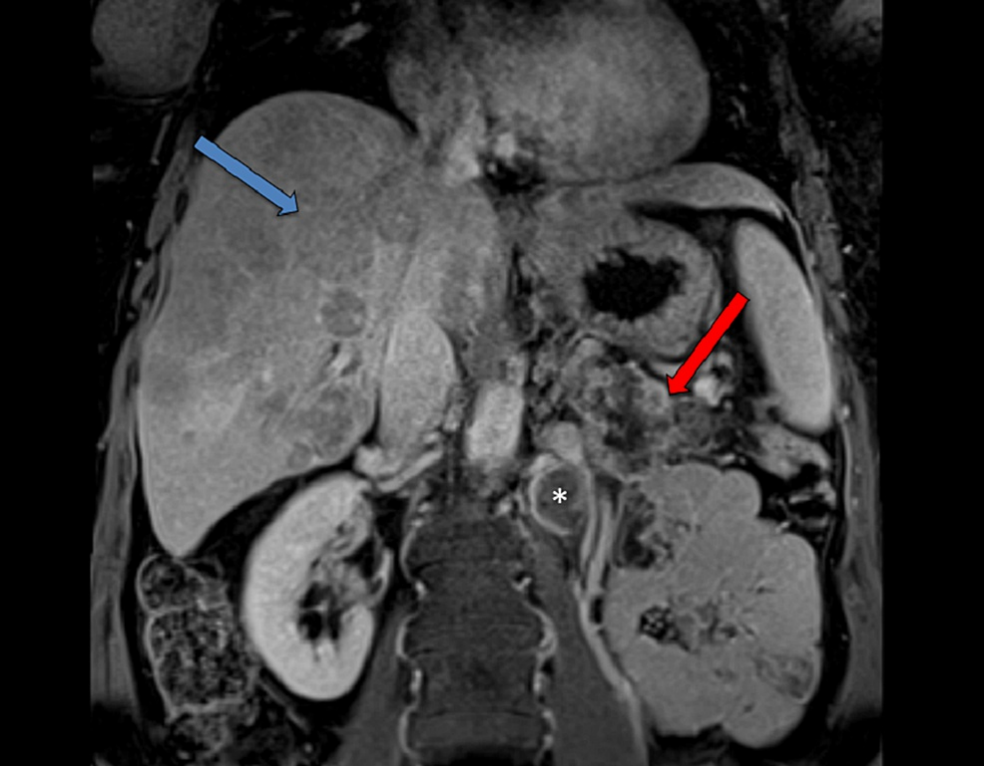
Magnetic resonance imaging (coronal) shows a pancreatic tail mass (red arrow), liver metastasis (blue arrow), and retroperitoneal lymphadenopathy (asterisk).
